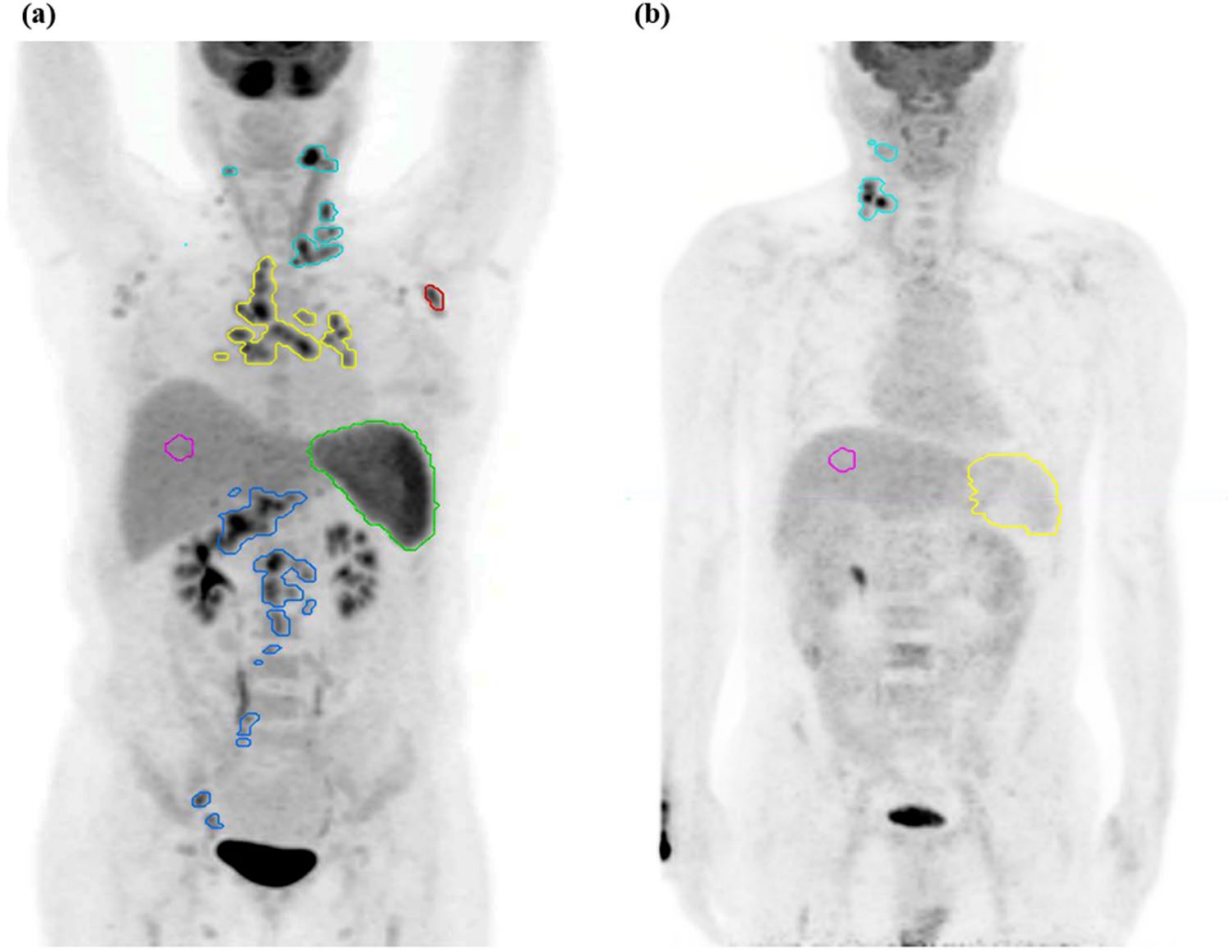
Maximum intensity projection images of representative mild and severe Kikuchi-Fujimoto disease patients with lesion segmentation. (a) A patient with severe Kikuchi-Fujimoto disease. Systemic increase in ^18^F-FDG uptake in the spleen and lymph nodes is seen. (b) A patient with mild Kikuchi-Fujimoto disease. Lesser FDG-avid lymph nodes are seen.
